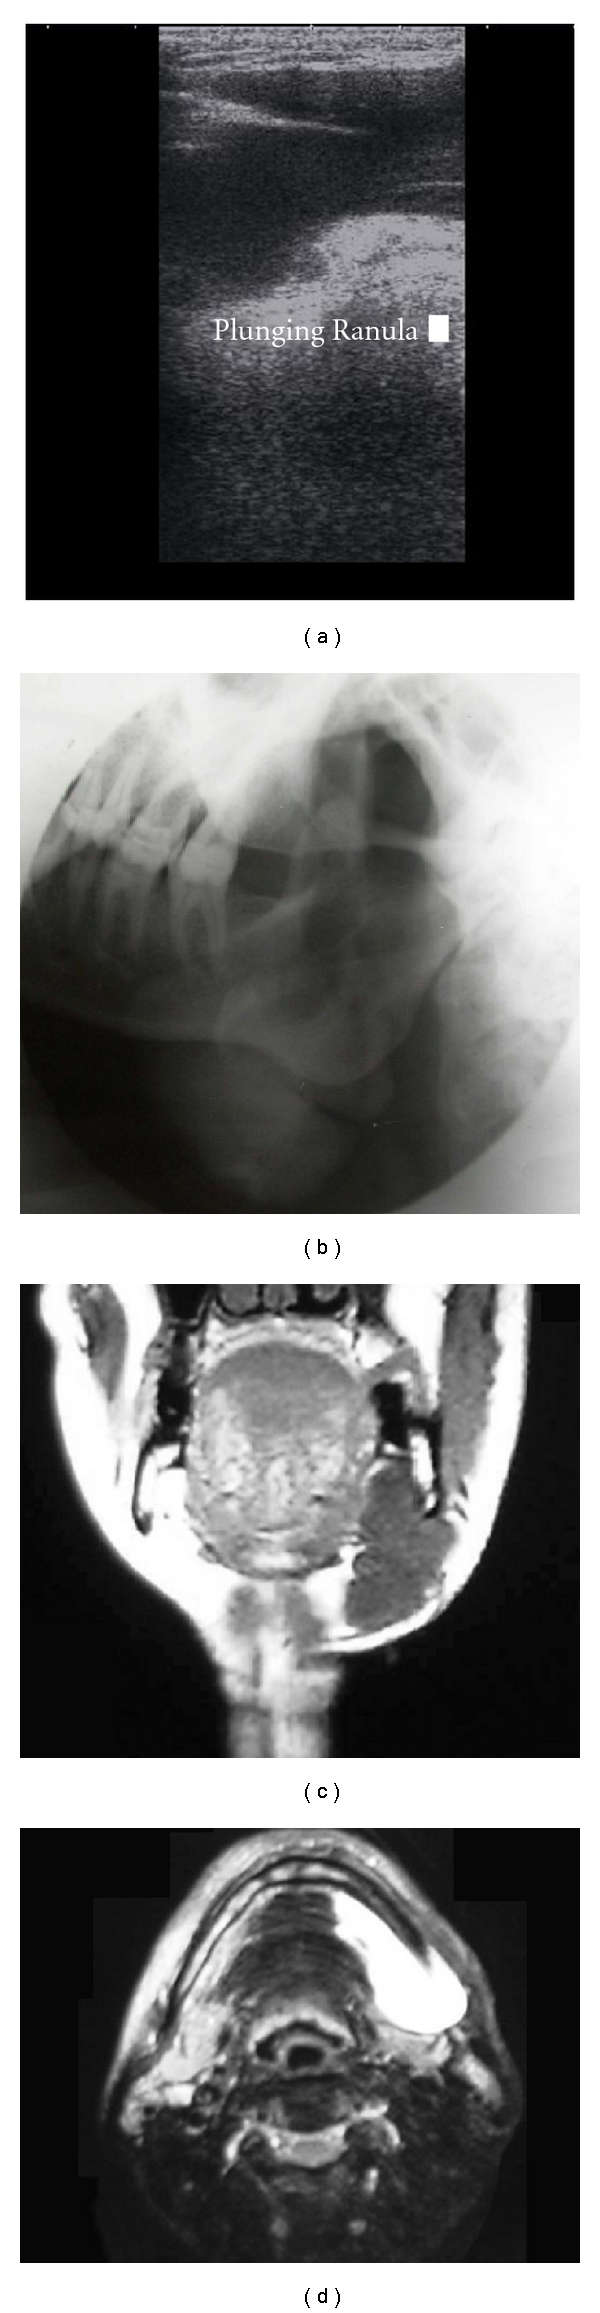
(a) Ultrasonogram showing anechoic fluid collection in left submandibular region. (b) Lateral oblique projection of submandibular region with contrast media injected in sublingual space. (c) Coronal T-1 weighed MR image showing hypointense area of fluid collection above and below the mylohyoid muscle. (d) T-2 weighed axial MRI section showing the extensions of the fluid collection.
